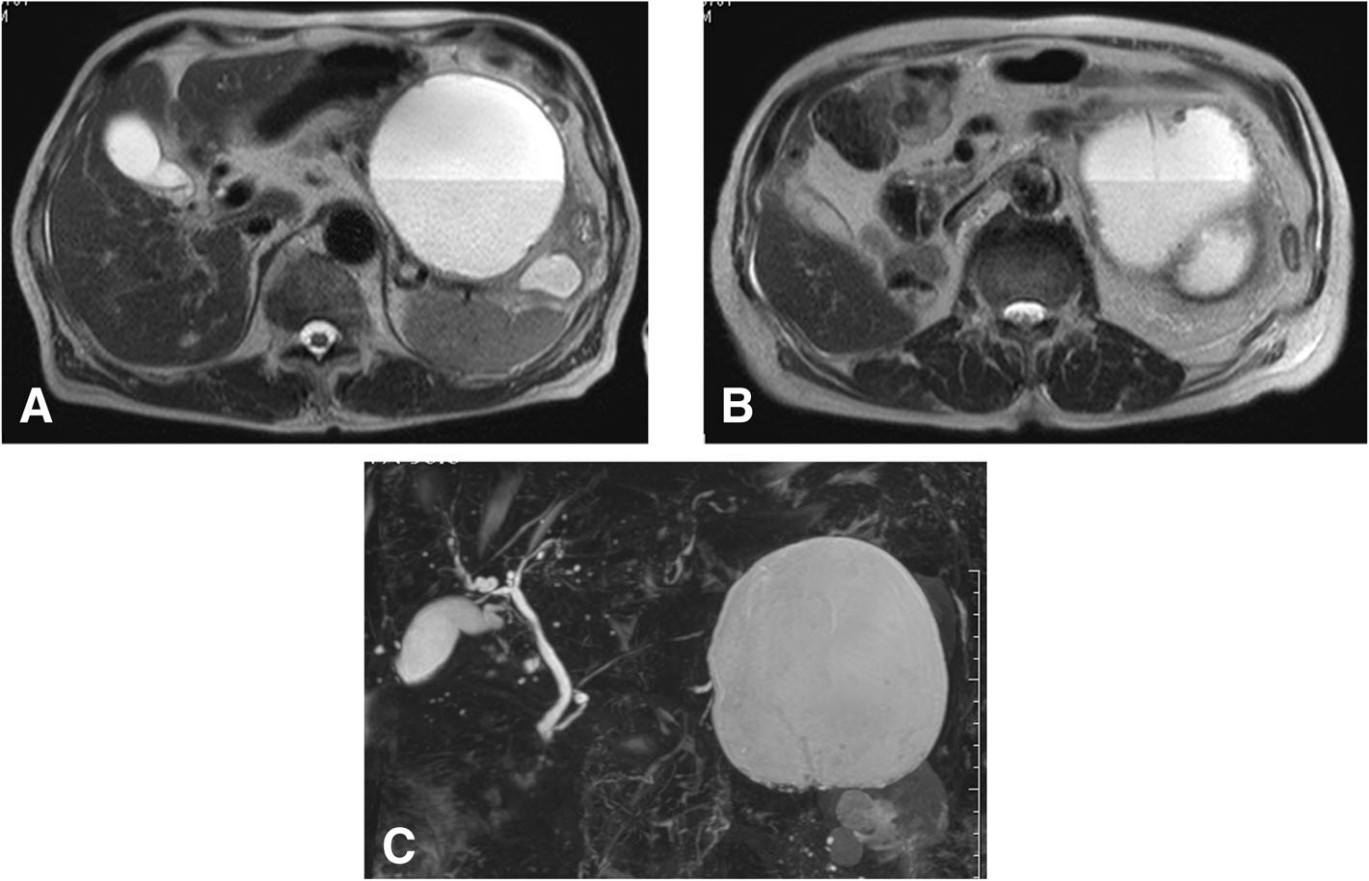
Magnetic resonance imaging (MRI) findings. MRI demonstrated a liquid surface in the pancreatic cyst (a, b). The cyst contained mucinous components suggesting infection. Magnetic resonance cholangiopancreatography did not show pancreatic duct dilation (c)
